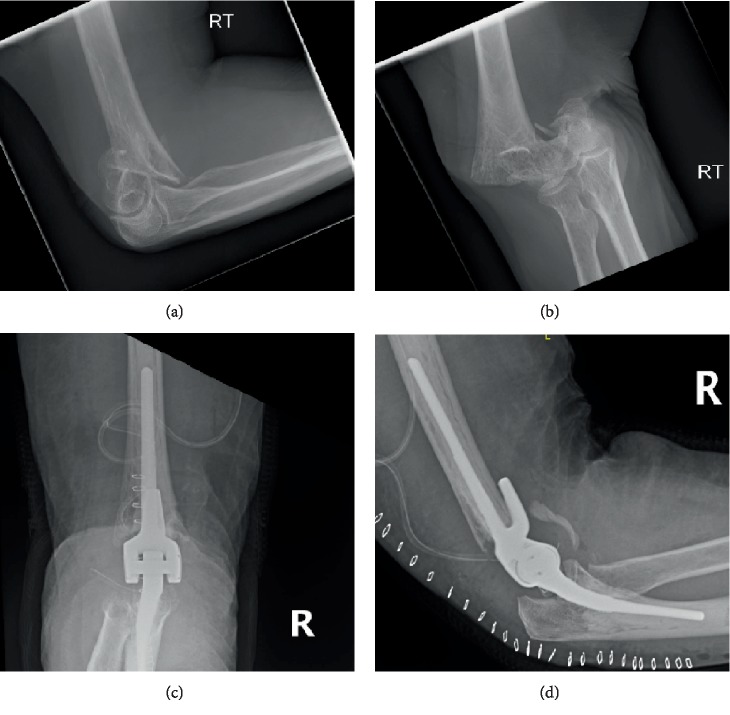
(a, b) Initial X-rays of right distal humerus fracture. (c, d) Immediate postoperative X-rays following total elbow arthroplasty.
